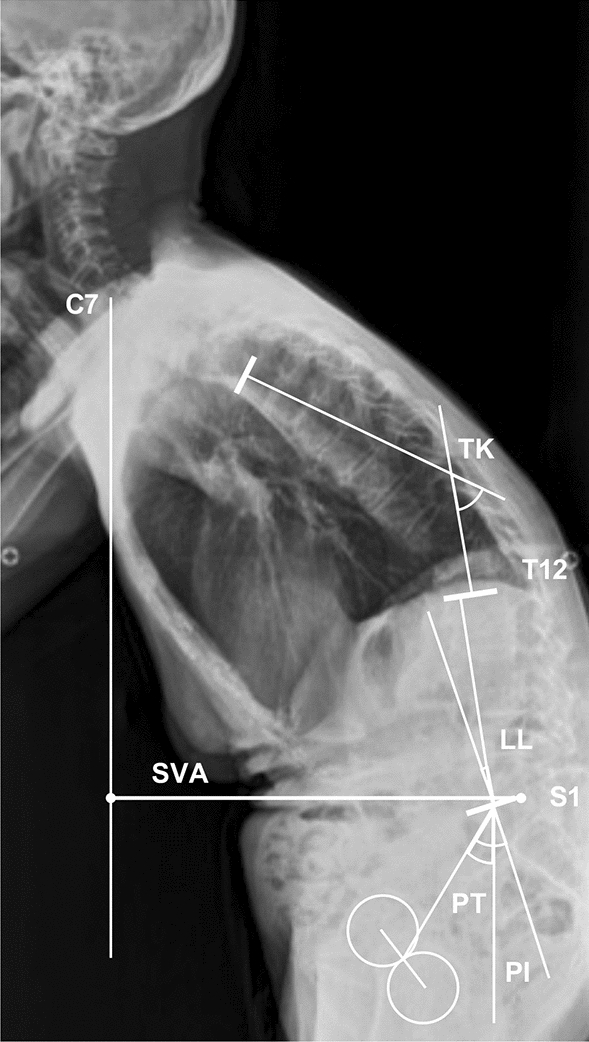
Static spino-pelvic parameters measured by full-spine lateral standing radiographs. SVA, the sagittal vertical axis; TK, thoracic kyphosis (T5-12); LL, lumbar lordosis (T12-S1); PT, pelvic tilt; PI, pelvic incidence.
